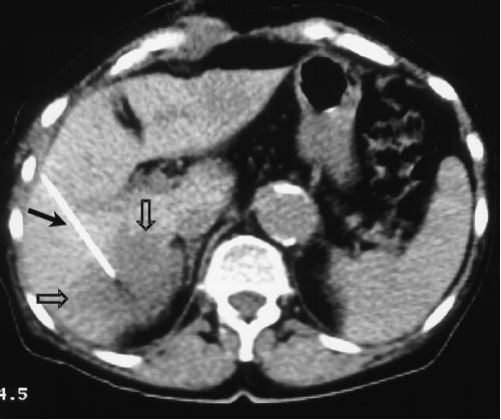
Visualization of the injection procedure in a hepatic metastasis (open arrows) shown in segment 5 using the CARE vision CT programme. Note the needle placed centrally in the tumour (arrow).
